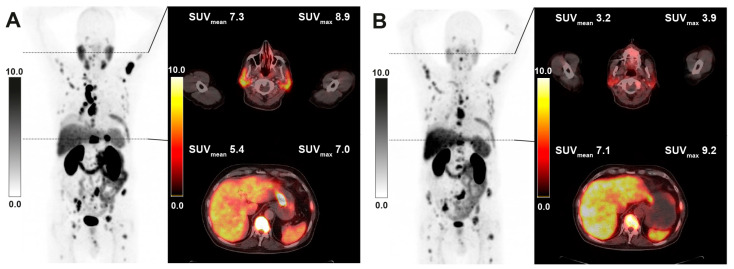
Sample [^68^Ga]Ga-PSMA-11 PET/CT images of a 64-year-old patient with multifocal metastatic disease receiving 2 cycles of RLT with cumulative 13.9 GBq of [^177^Lu]Lu-PSMA-617. Maximum intensity projection (MIP) images (left) with axial fusion images showing the parotid gland and liver uptake at baseline (A) and after RLT (B).
